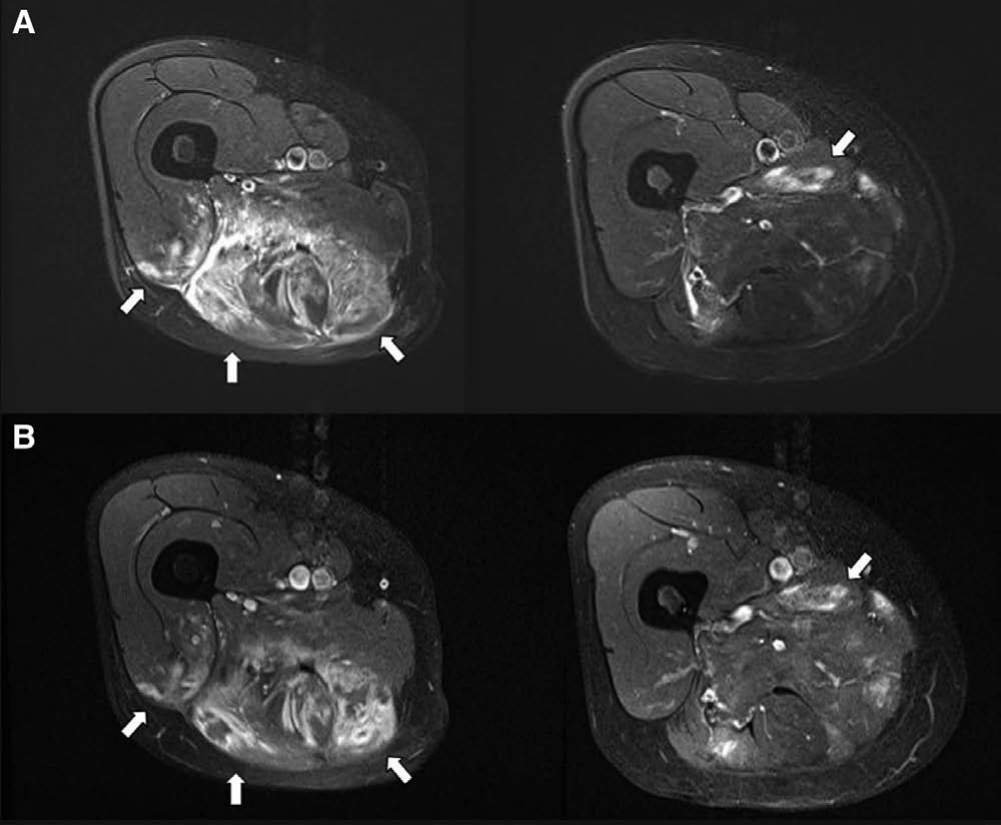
Initial MR images of thigh in a patient with polyarteritis nodosa. (A) Axial fat-suppressed T2-weighted images show diffuse high signal intensity in the biceps femoris, semitendinosus, semimembranosus, and partial involvement in vastus lateralis and vastus intermedius (arrows, left image). High signal intensity is also observed in adductor longus and adductor brevis muscles (arrow, right image). (B) Axial contrast-enhanced fat-suppressed T1-weighted images show diffuse enhancement of the affected muscles (arrows).
